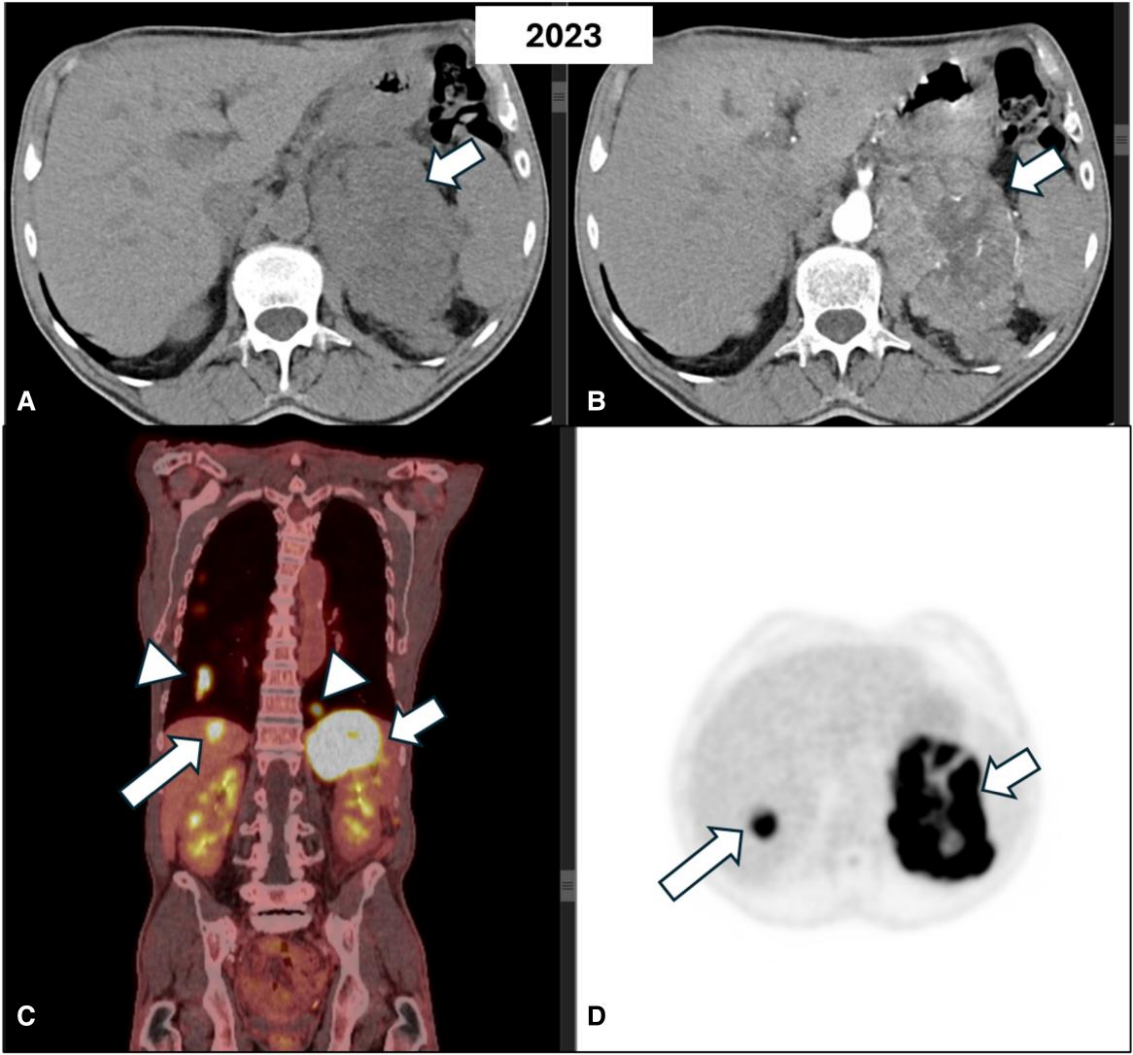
Axial computed tomography of the abdomen of the same patient before (A) and after (B) intravenous contrast administration shows a heterogeneous mass with central necrotic foci involving the left adrenal gland and extending to the surrounding tissues (arrows). On ^18^FDG-PET images (C-D), the lesion demonstrates avid ^18^FDG uptake (small arrow) and high metabolic activity lesions in the liver (long arrow) and the lungs (arrowheads) suspicious of metastases. Abbreviations: ^18^FDG-PET, 18F-fluorodeoxyglucose positron emission tomography.
